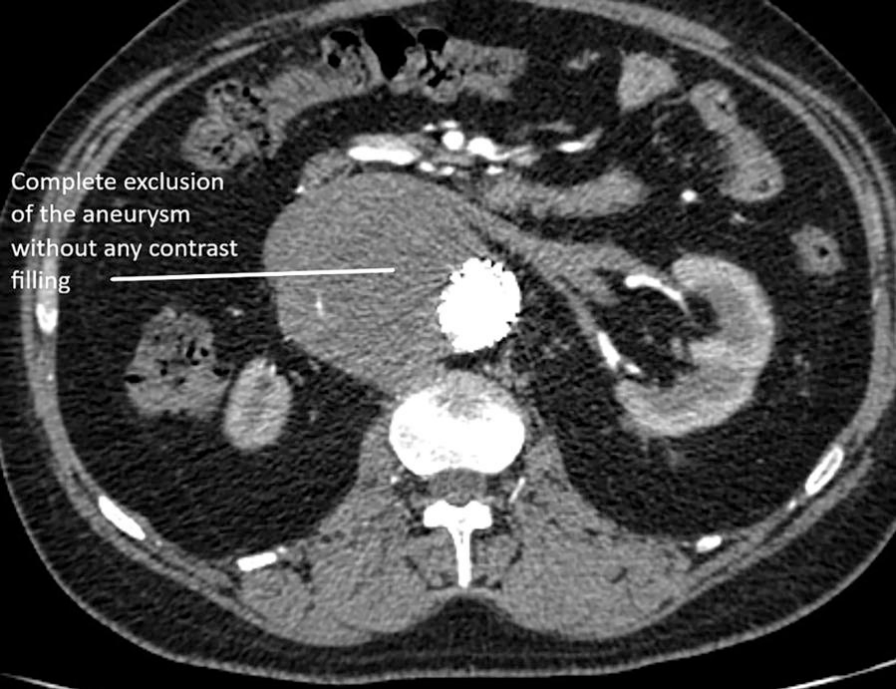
Follow-up CT. Complete obliteration of the saccular aneurysm in the abdominal aorta.
